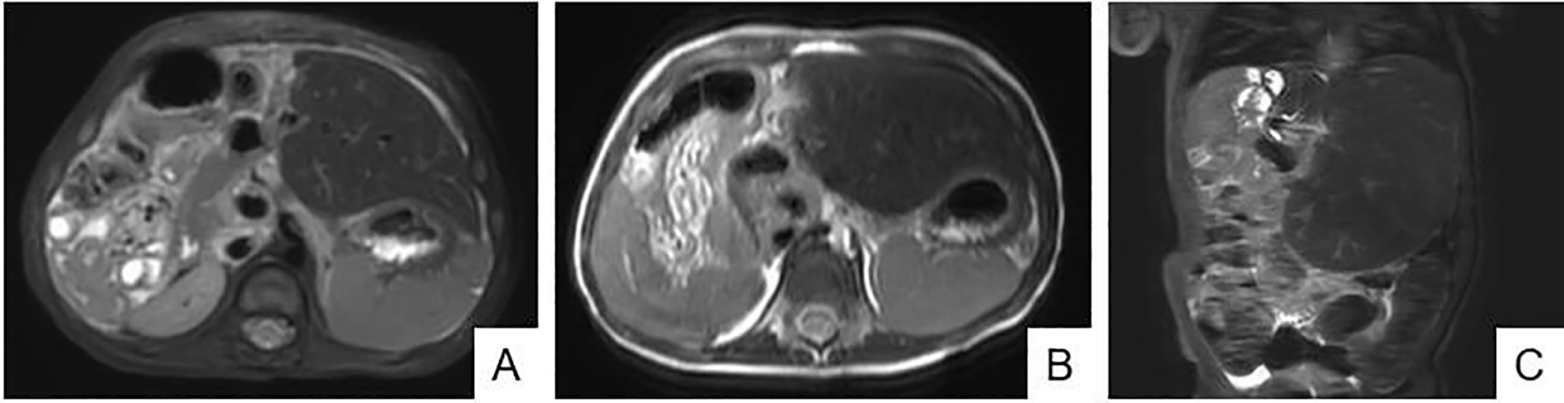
Postoperative Follow-up MRI. Follow-up results at 1 month (A) and 6 months (B, C) postoperatively show the remaining liver is well-preserved, with no tumor recurrence or metastasis to other sites.
